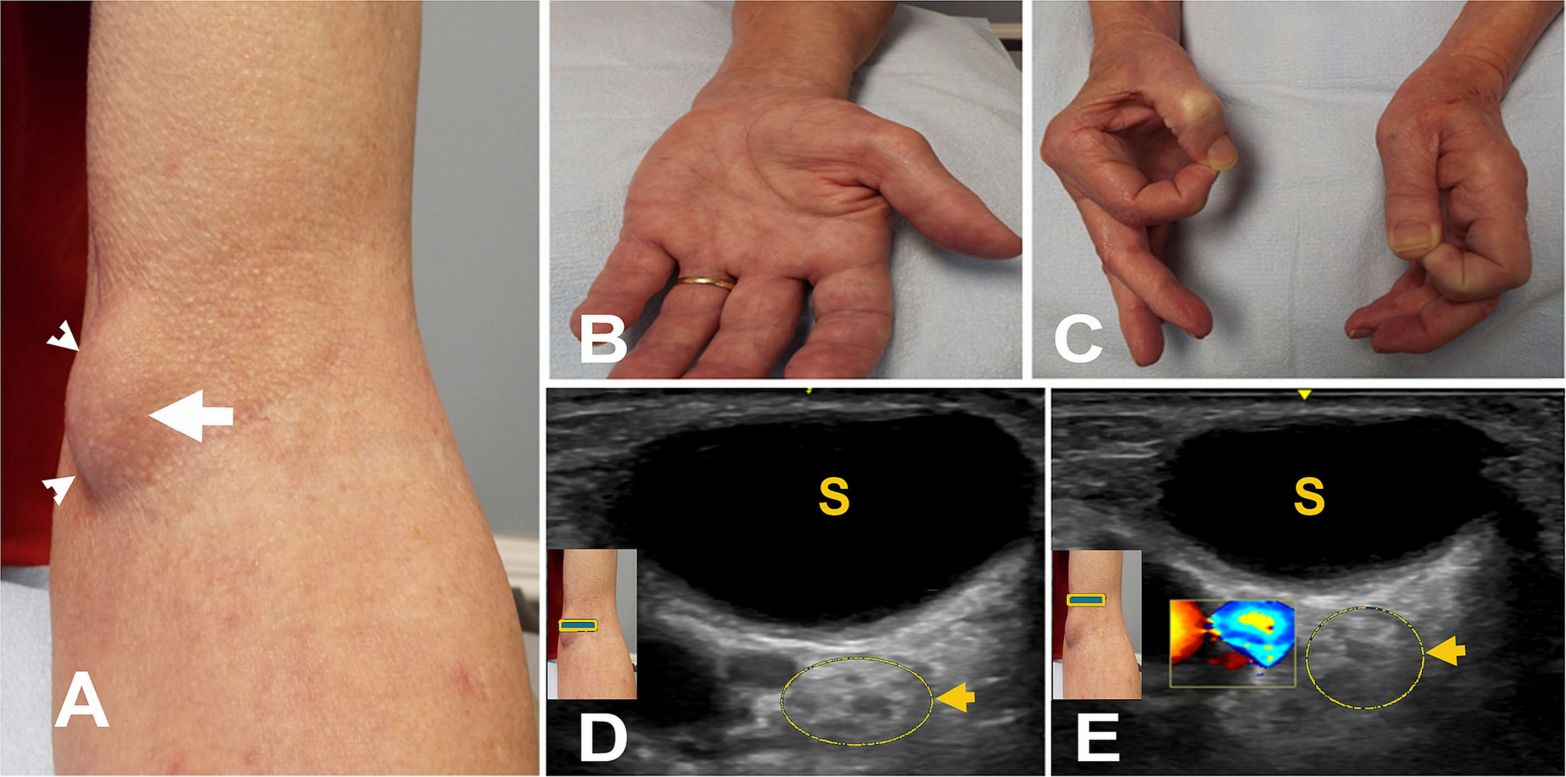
(A) Mass after brachial artery cannulation (arrowheads). (B) Atrophy of left thenar muscles. (C) Positive O sign on the left. (D) Short axis view at the elbow with color Doppler over the mass (square box) showing no blood flow. The arrow points to the hyperechoic enlarged median nerve with a few fascicles. (E) Short axis view with color Doppler (square box) 5 mm proximal to “A” showing the brachial artery with hyperechoic enlarged median nerve with a few fascicles (arrow). S, seroma.
